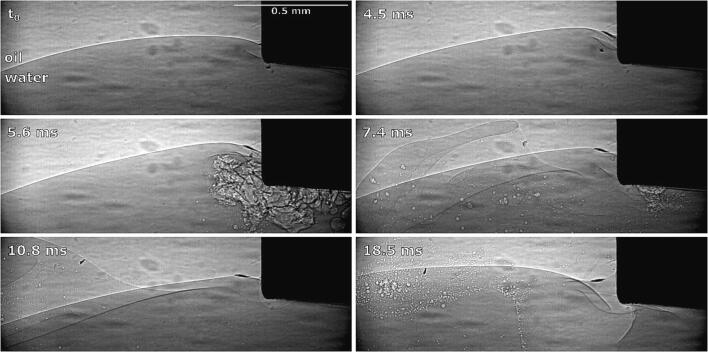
Synchrotron observations of brief emulsion formation at ultrasonic horn’s corner and downwards interface movement.
